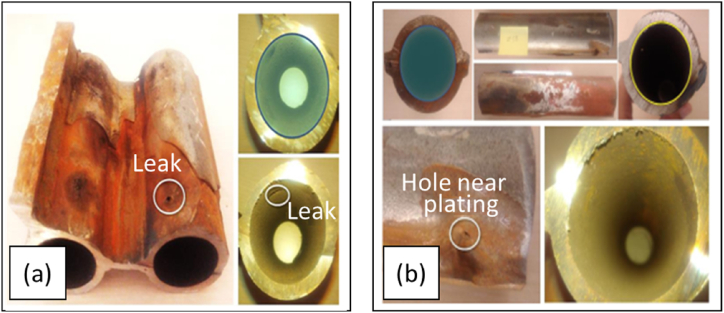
Surface macro photos of a) WT, b) CTA.
